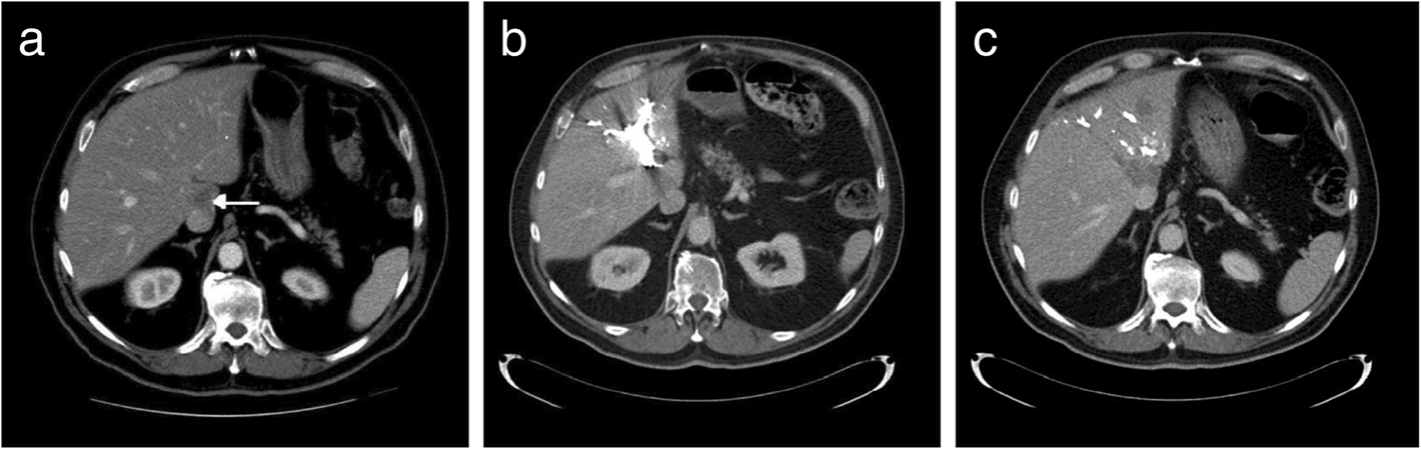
CT scans showing portal vein embolization and left and middle hepatic vein embolization a) CT scan showing metastatic lesion (arrow), b) CT scan after portal vein embolization, c) CT scan after left and middle hepatic vein embolization.
